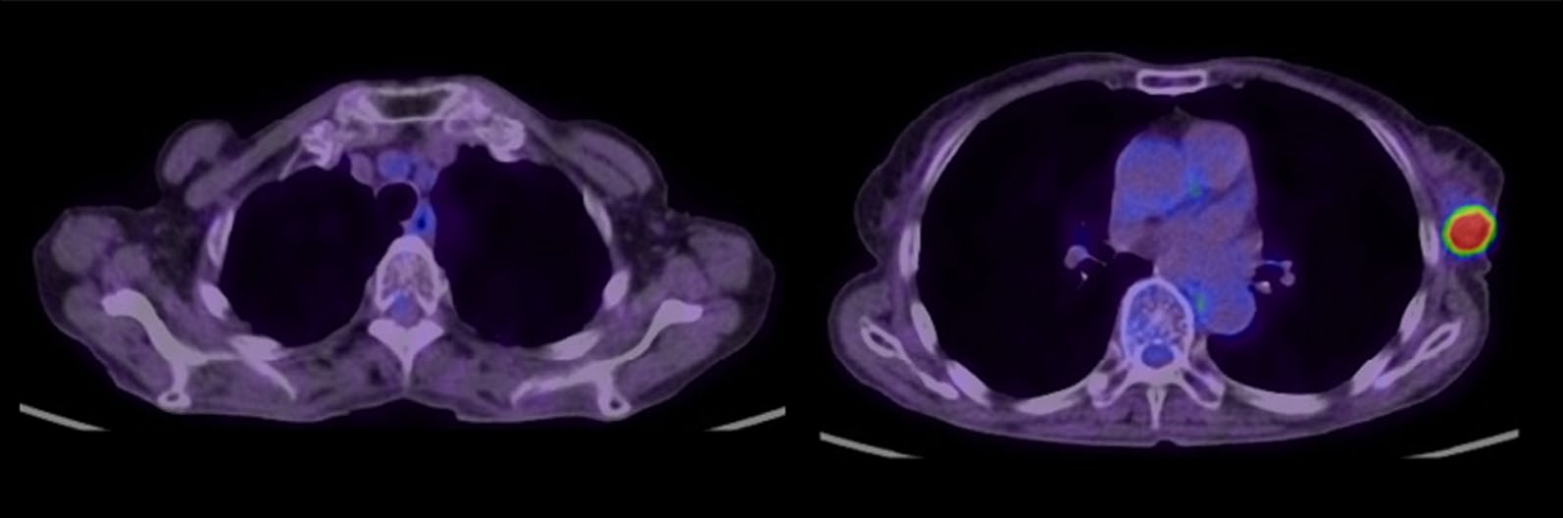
PET–CT scan findings. Left: no lymph node metastasis is suspected. Right: abnormal accumulation is only seen in the left breast
